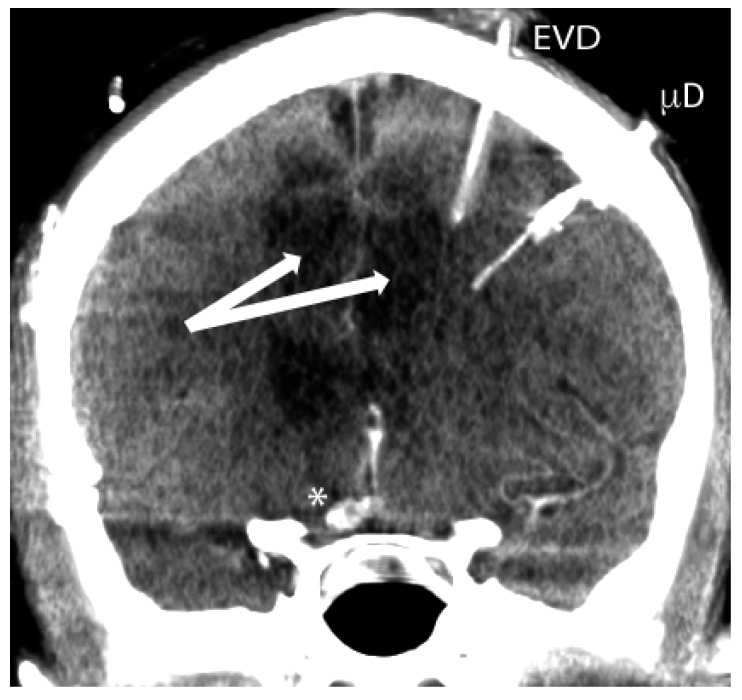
Computed tomography showing External ventricular drain (EVD) and microdialysis (µD) probe in place in aSAH patient. Sometimes the multimodality monitoring probes are in proximity to injured brain, but often they are not. Severe vasospasm resulting in ischemic brain (arrows) occurred days after anterior communicating artery aneurysm clipping (*).
